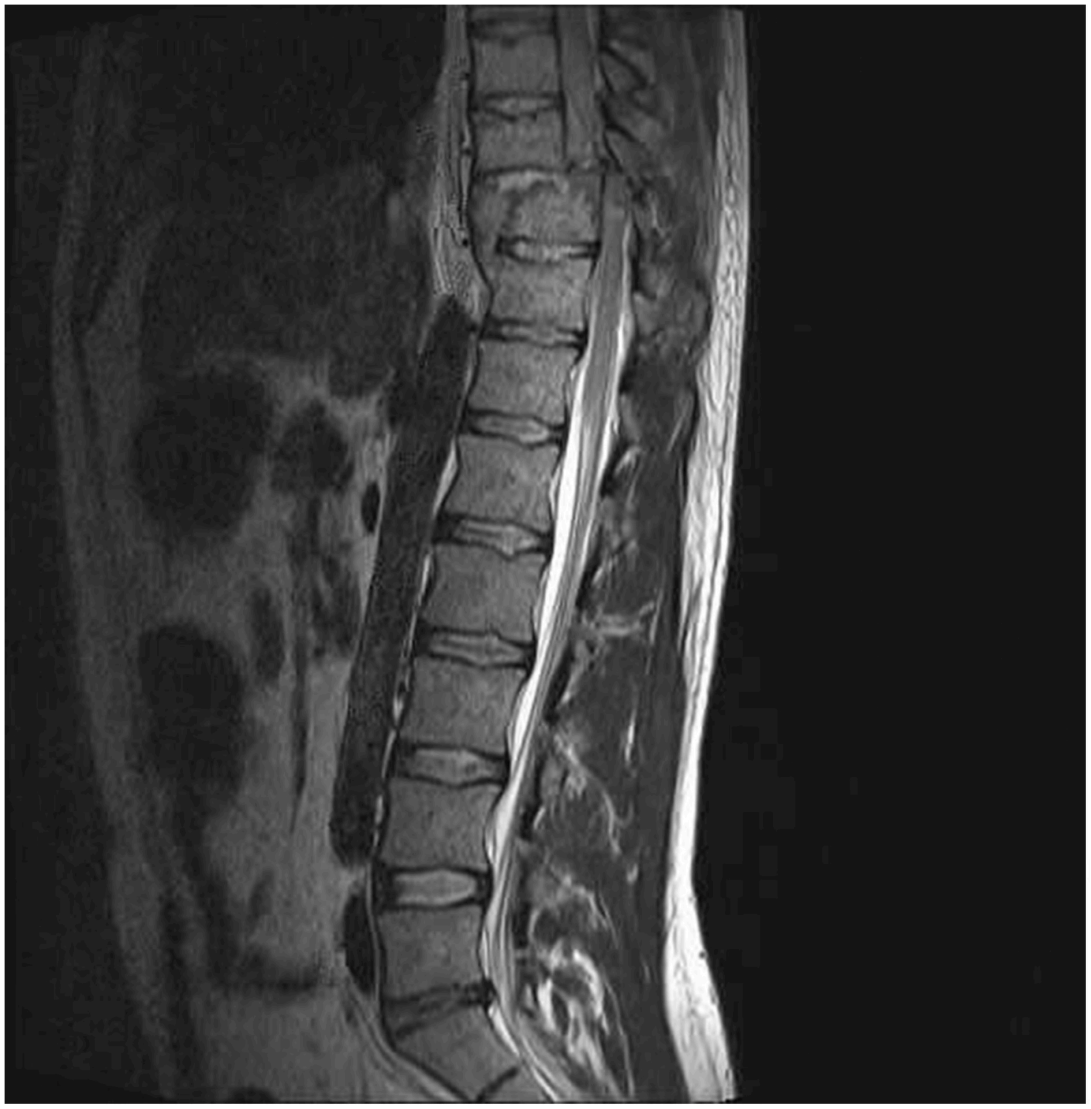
MRI sagittal image of included patient with complete spinal cord transection. All included spinal cord injured patients had MRI evidence of complete spinal cord transection. This image was obtained from a 25 year-old male with a T10 complete neurological deficit.
